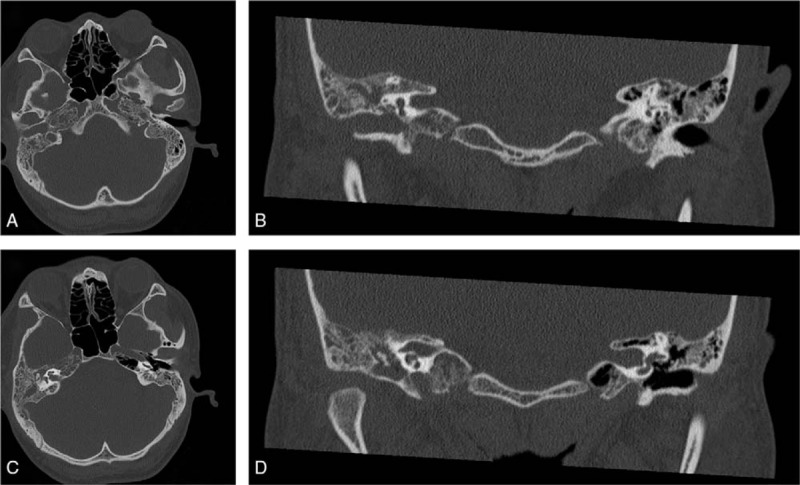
Axial (A) and coronal (B) computed tomography (CT) images showed a soft-tissue density occupying the right external auditory canal. Axial (C) and coronal (D) CT images showed occupation of the right mastoid antrum, tympanic cavity, embedment of the right ossicle, and no significant temporal bone destruction.
